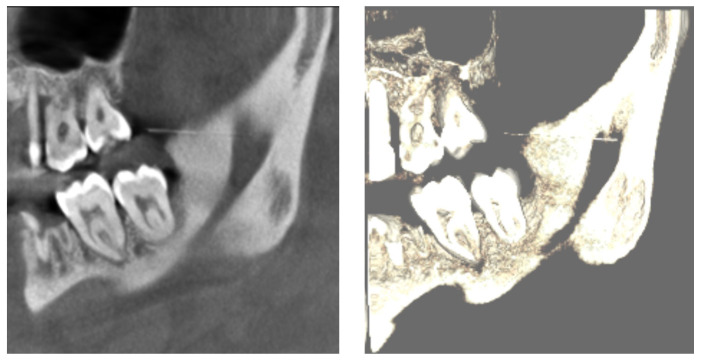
Multiplanar CBCT (panoramic) reconstructions highlighting depth and localization.
